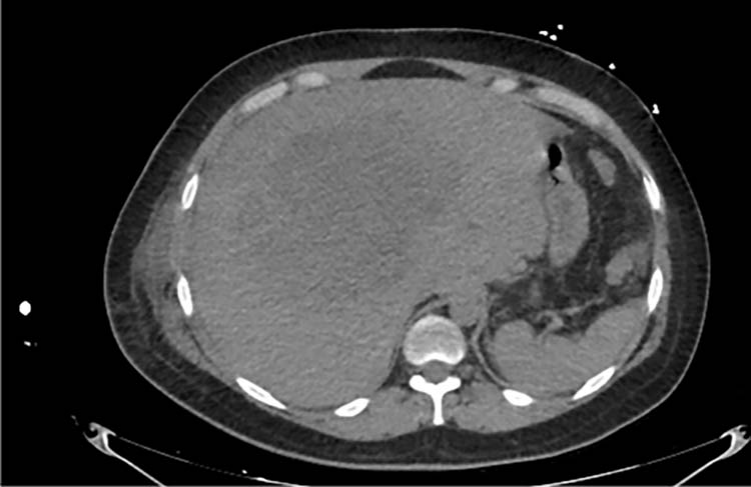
Axial abdominal CT at admission: 20 cm nodular lesion occupying the right liver lobe.
